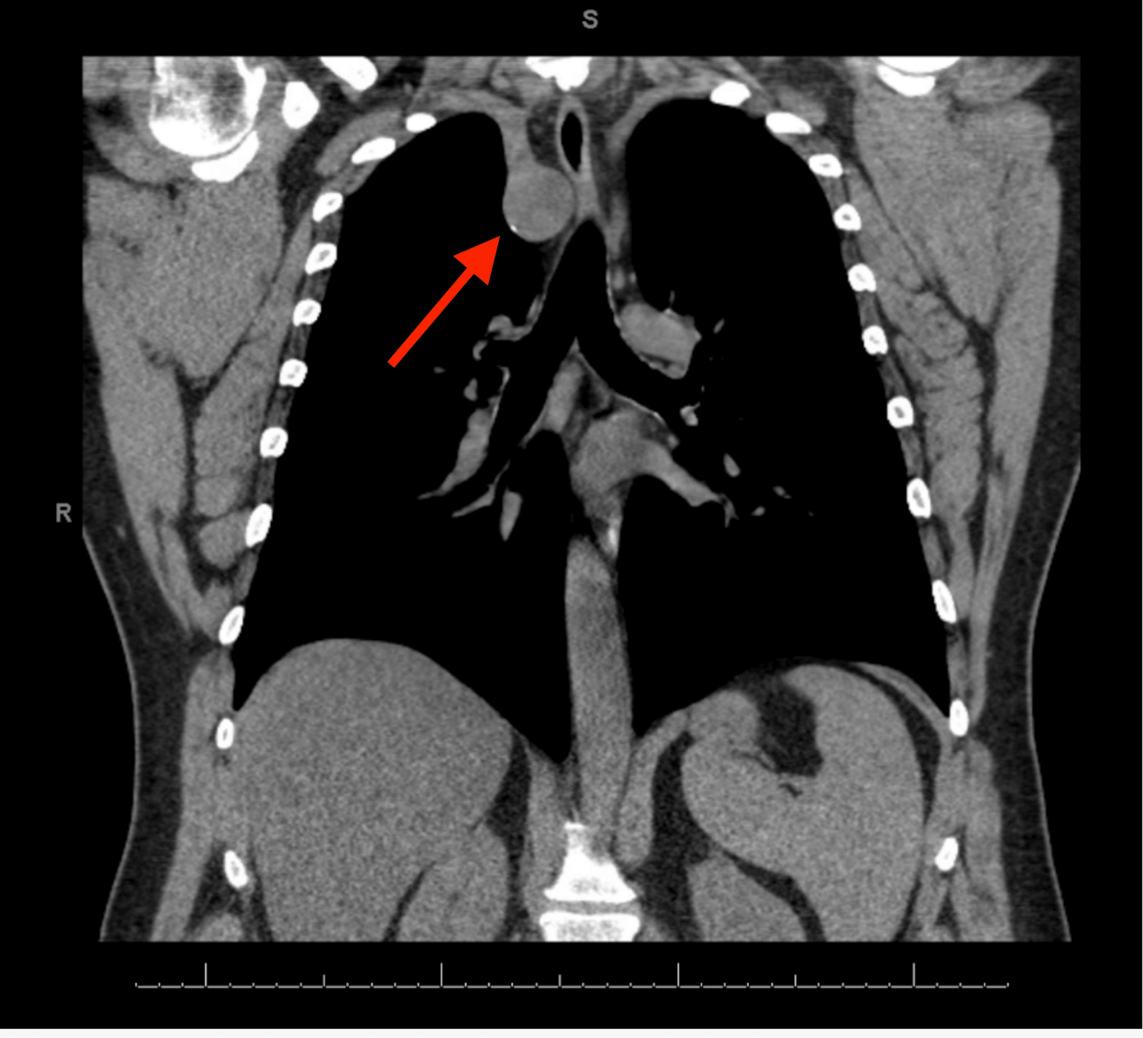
Coronal CT image from 2025 demonstrating a right-sided aortic arch (red arrow) with significant tracheal compression. CT: computed tomography
